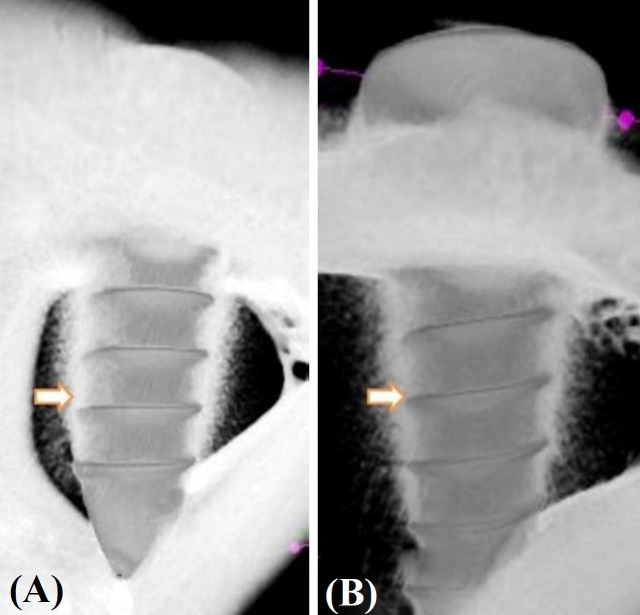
µ-CT images of the (A) control and (B) experimental Ti screws. Arrows show the newly formed bone tissue
